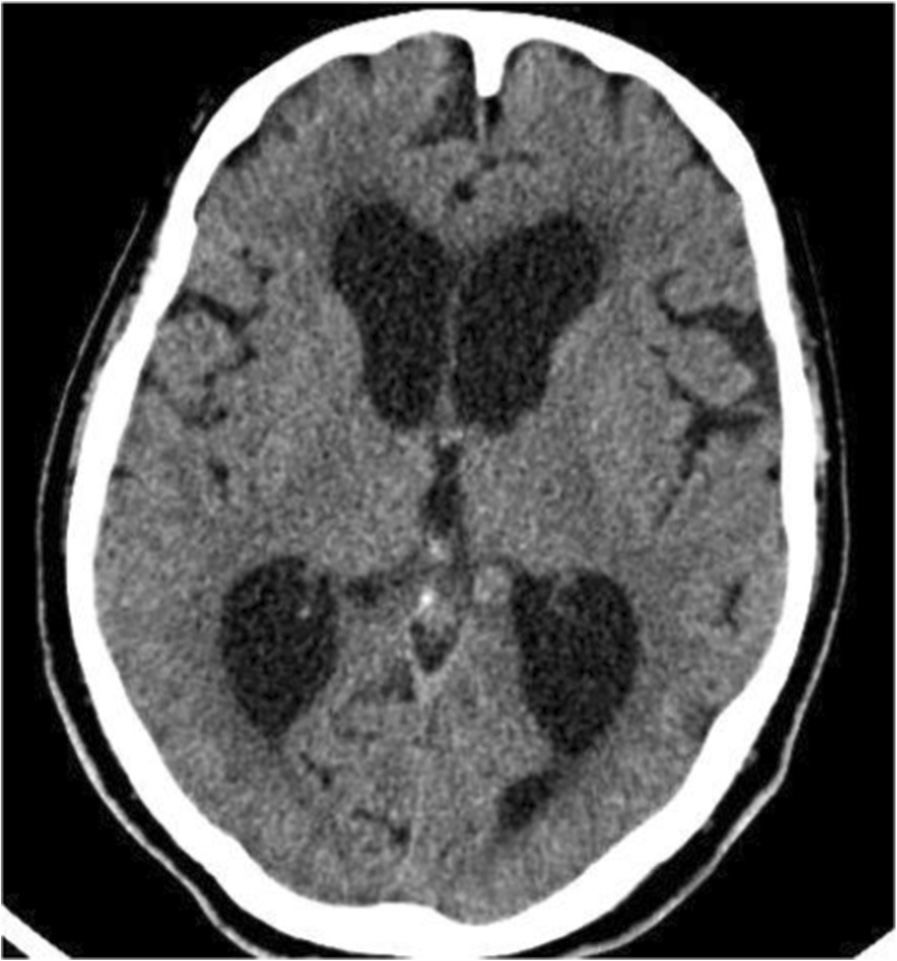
Brain computed tomography scan showing marked ventricular dilatation without calcification.
